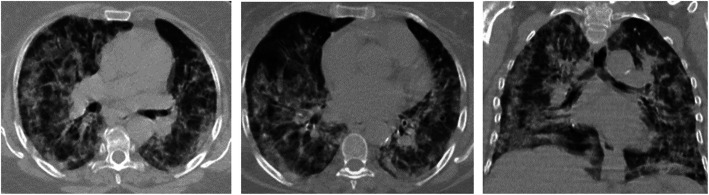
Male patient, 60 years old, was complaining of dry cough and dyspnea and was confirmed to be COVID-19 by RT-PCR. These axial and coronal non- contrast CT chest images (lung window) show typical findings with high suspicion of COVID-19 pneumonia in terms of diffuse multifocal ground-glass opacities involving both lungs with CORADS-3. Both upper and lower lobes have a score of 5 (with ≥ 75% involvement), while the middle lobe has a score of 4 with (50% to < 75 % involvement), so the total CT lung severity score is 24 with 96% of lung involvement denoting severe grade of lung affection
